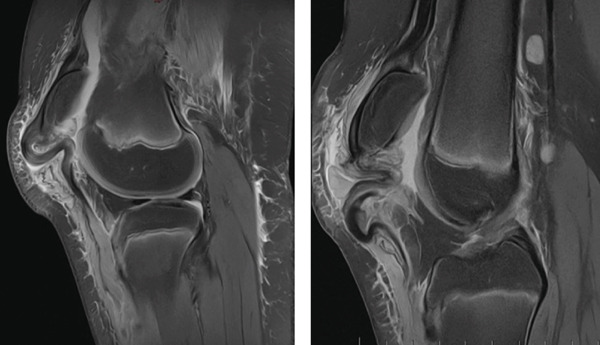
Right and left knee MRI showing purely tendinous distal patellar tendon avulsion.
